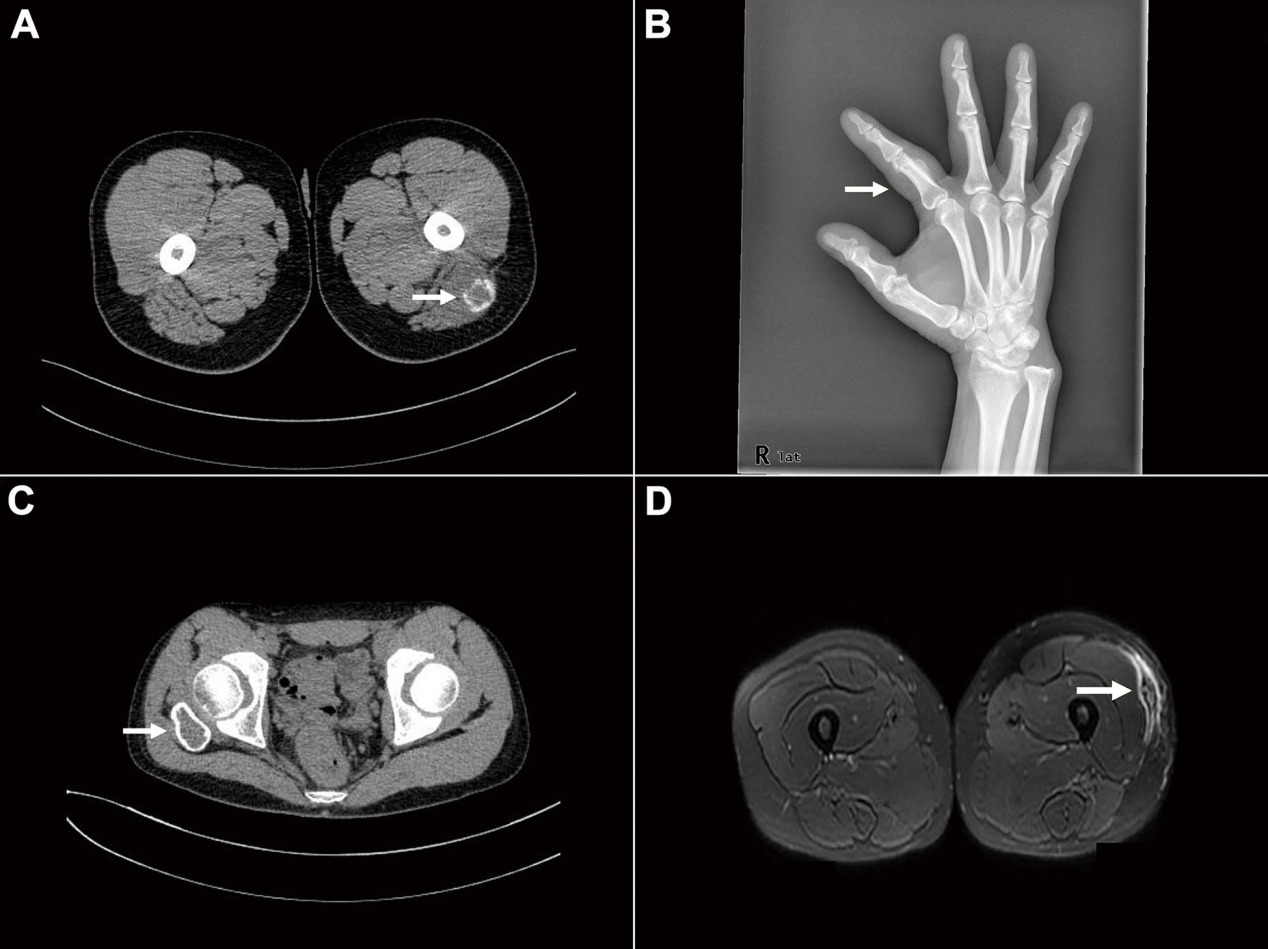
Imaging findings of *USP6*-associated soft tissue tumors with osteoid tissue. (A) CT showed a soft tissue density mass in the muscle, with well-defined boundaries and circumferentially circular density (arrow) (Case 33). (B) X-rays demonstrated a soft tissue mass in the right finger with calcification (arrow) (Case 54). (C) CT showed mixed-density shadows with clear boundaries around the right hip (arrow) (Case 60). (D) MRI showed mixed signals in the subcutaneous fat layer of the left thigh, with clear boundaries (arrow) (Case 63).
